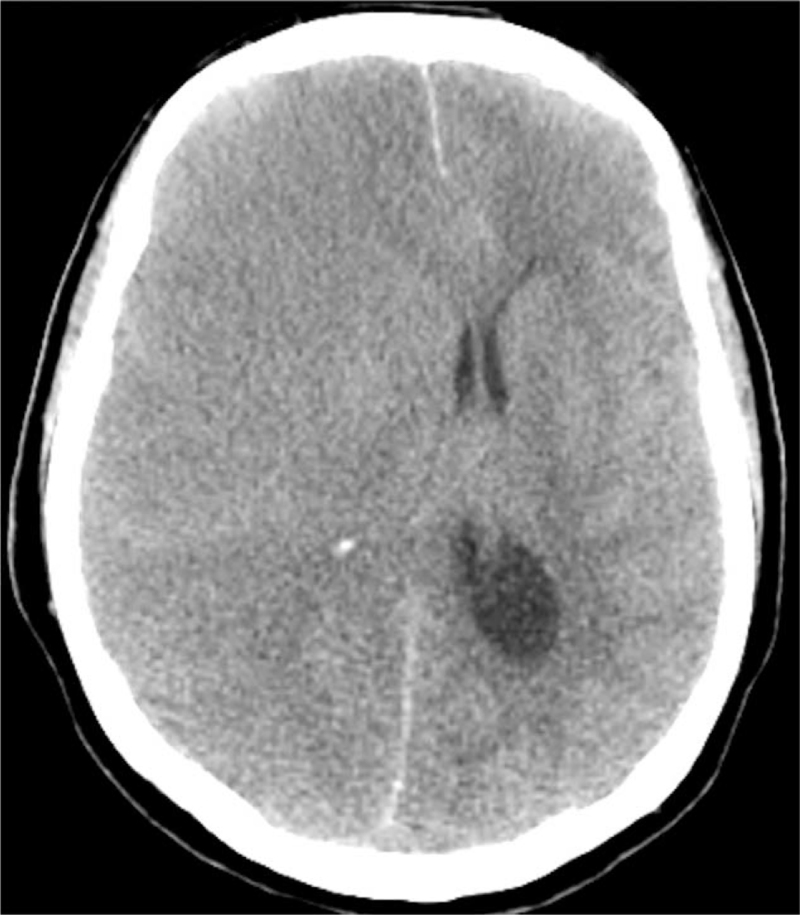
Follow-up brain computed tomography taken after 2 days shows a marked increase in the extent of infarct-related edema in the right cerebral hemisphere with left-sided midline shifting.
